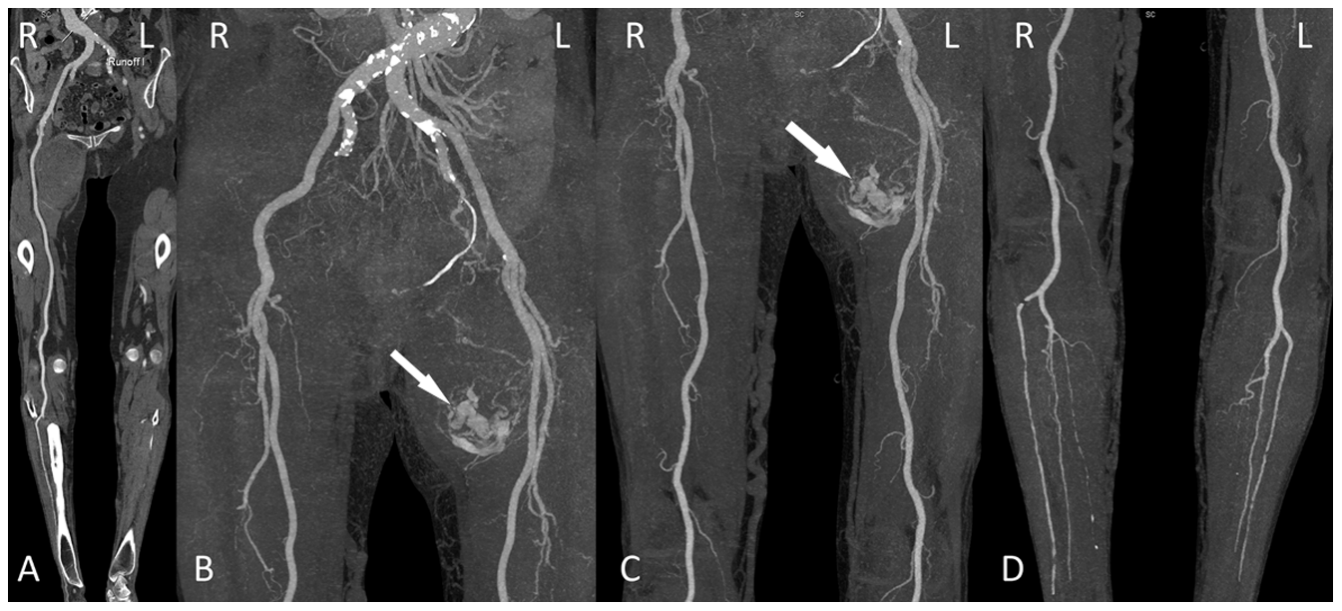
Lower extremity CTA using 70-year-old woman with tumor. A, curved planar reformatted image and B–D, maximum intensity projection images. The image quality was visually classified as score 3 (good) in the aortoiliac (B), femoropopliteal (C) and lower leg segments (D). Note abnormal hypervascular mass (myxofibrosarcoma) in the left thigh (arrow in panels B and C).
